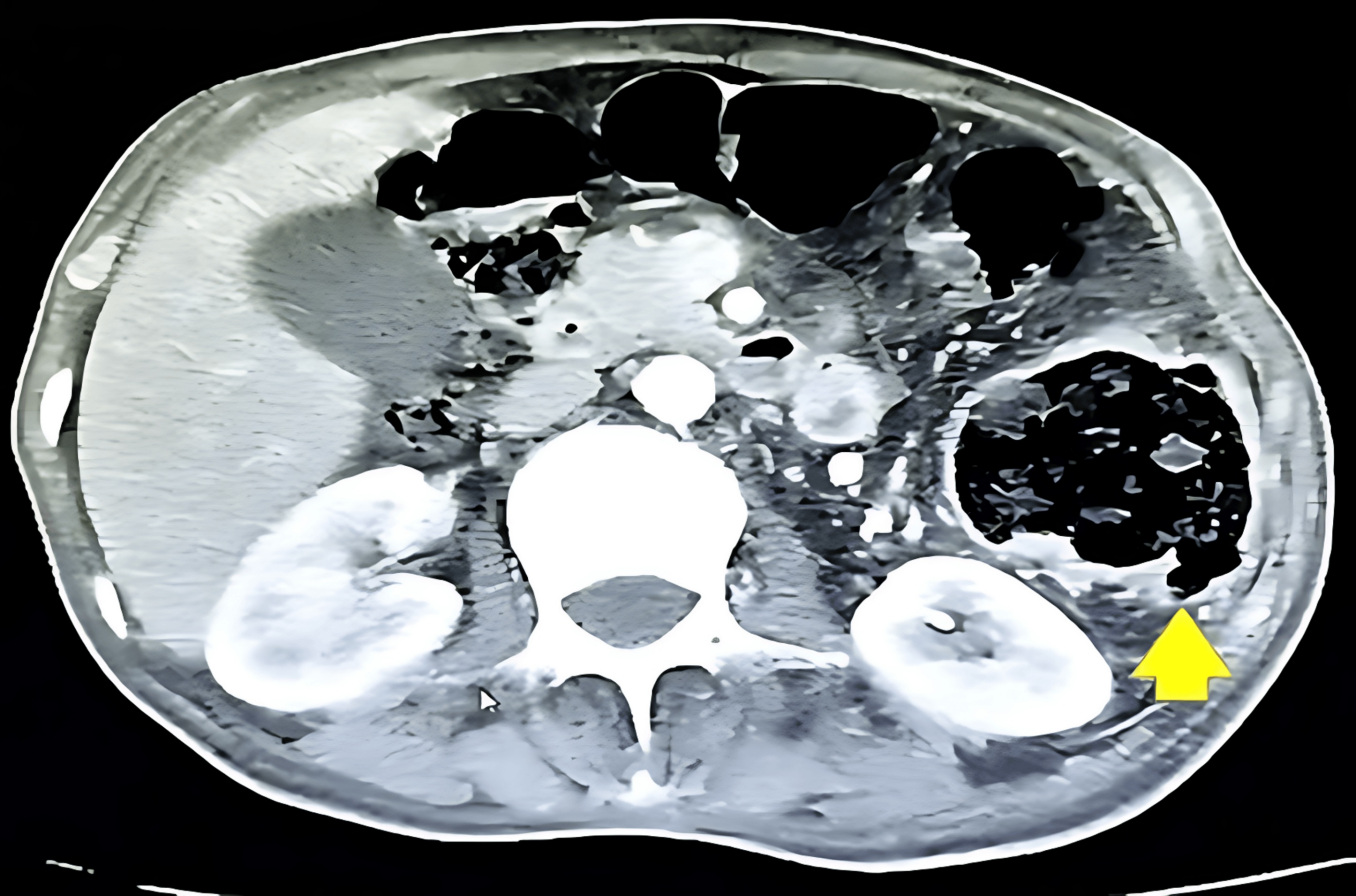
Axial CT scan showing a large lumbar abscess. Large hypodense fluid collection (yellow arrow) extends from the left lower quadrant to the posterior lumbar region, consistent with a complex abscess measuring approximately 20 mm in thickness and 200 mm in length.
